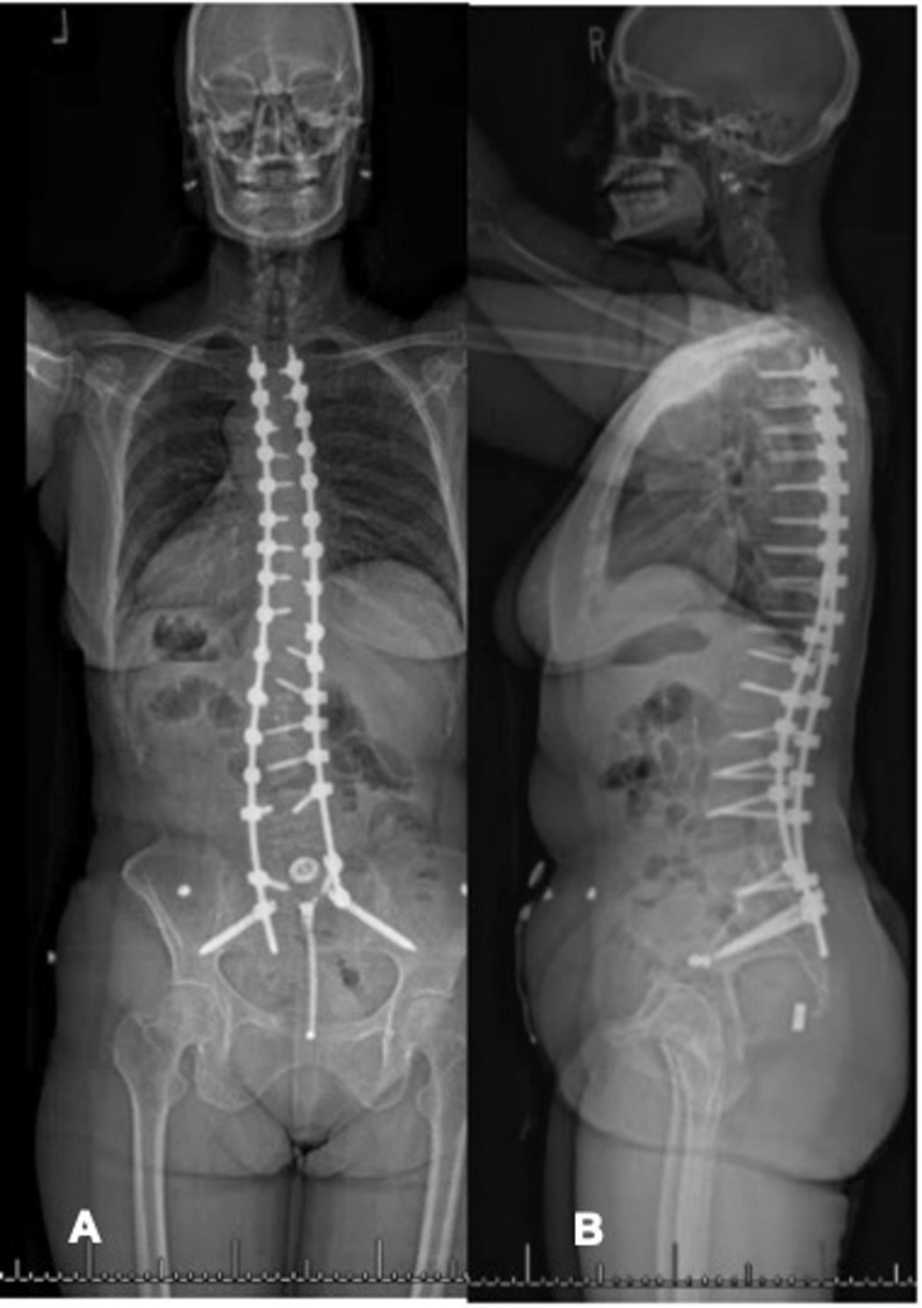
(A) AP and (B) lateral scoliosis X-ray status post T3-pelvis instrumentation and fusion. Post-operative sagittal parameters: thoracic kyphosis: 25°, lumbar lordosis: 31°, T1 pelvic angle (T1PA): 16°, pelvic incidence (PI): 50°, sagittal vertical axis: 0 cm, PI - lumbar lordosis (PI-LL) = 19°.
